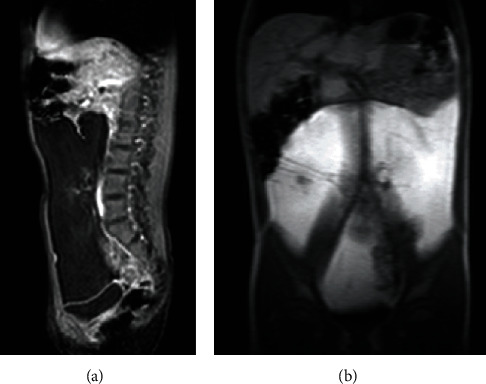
(a, b) MRI ((a) T1 weighted, (b) Thrive sequences) showing the mass occupying the entire abdominal cavity, compressing and displacing the bowel, the inferior vena cava, and common iliac veins.
